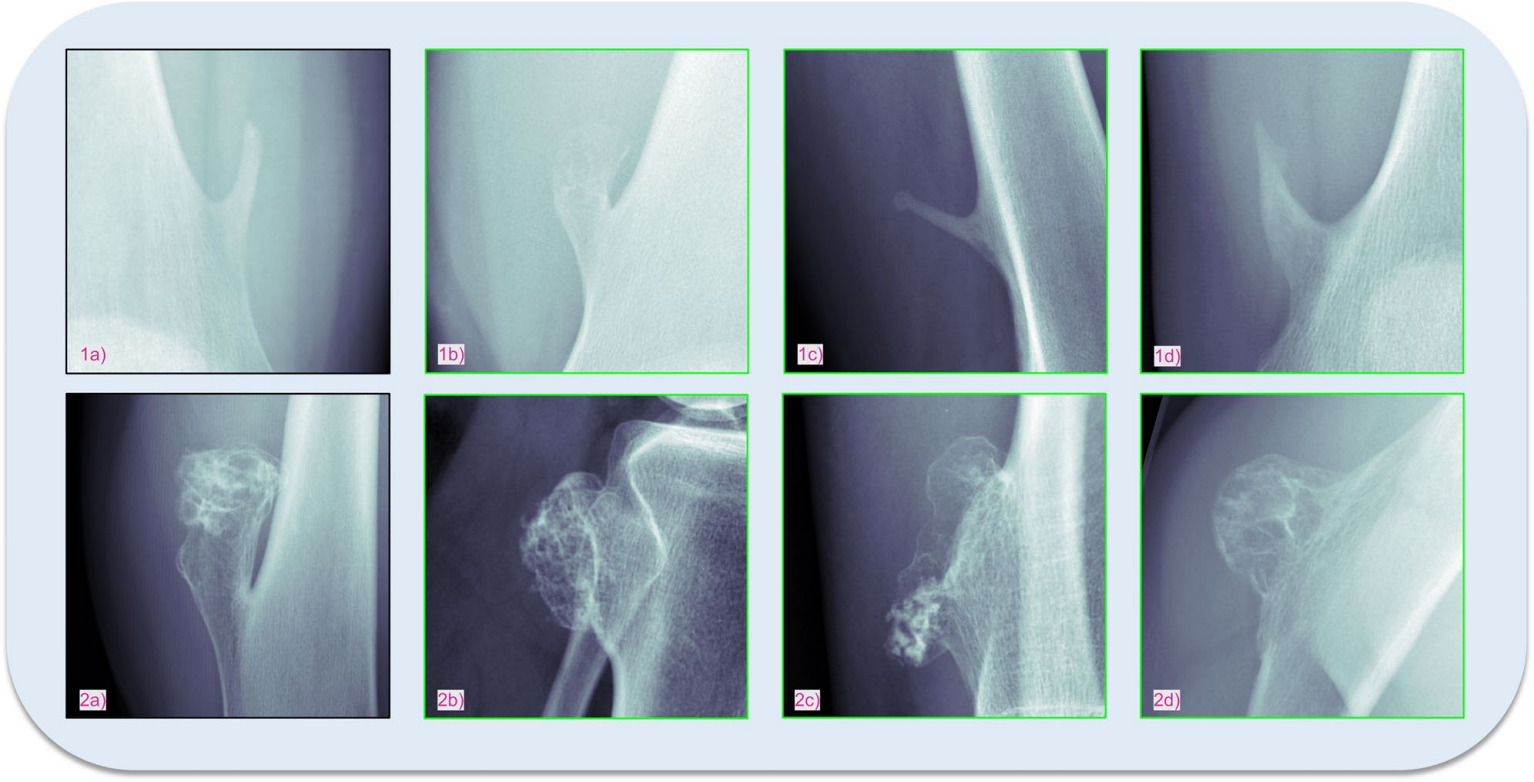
Examples of osteochondroma X-rays showcasing the model’s ability to accurately cluster different appearances of the same tumour entity. The target image is marked with a black frame, while correctly matched images are highlighted with a green frame
